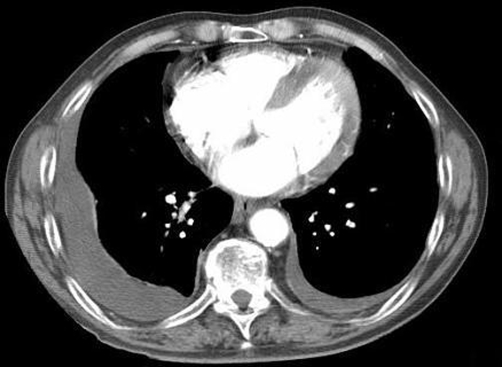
Computed tomography of the chest showing bilateral pleural effusions without other significant thoracic disease.
